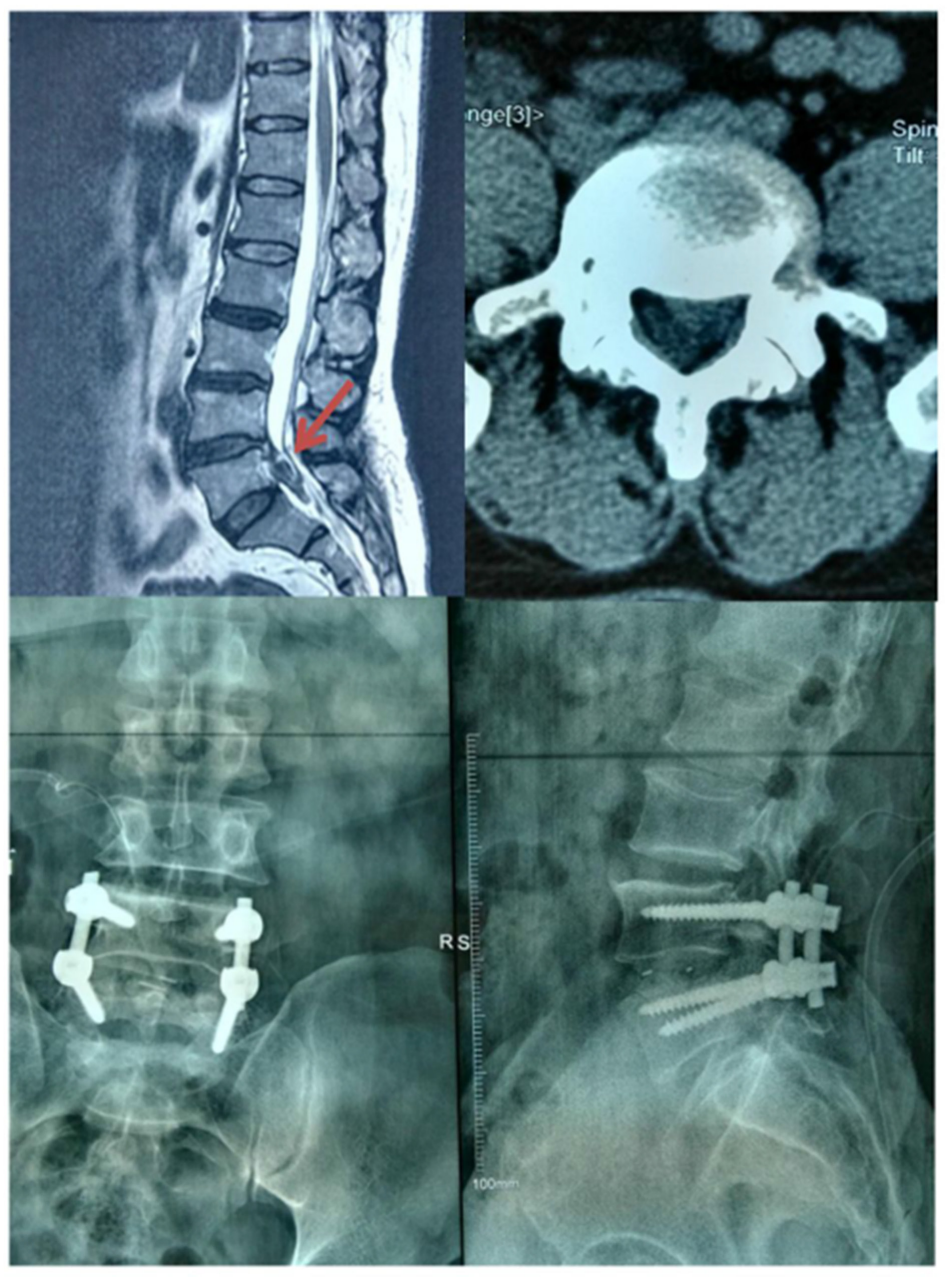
The prolapsed disc was removed from spinal canal after an emergency operation performed And an X-ray radiograph was taken postoperatively.
